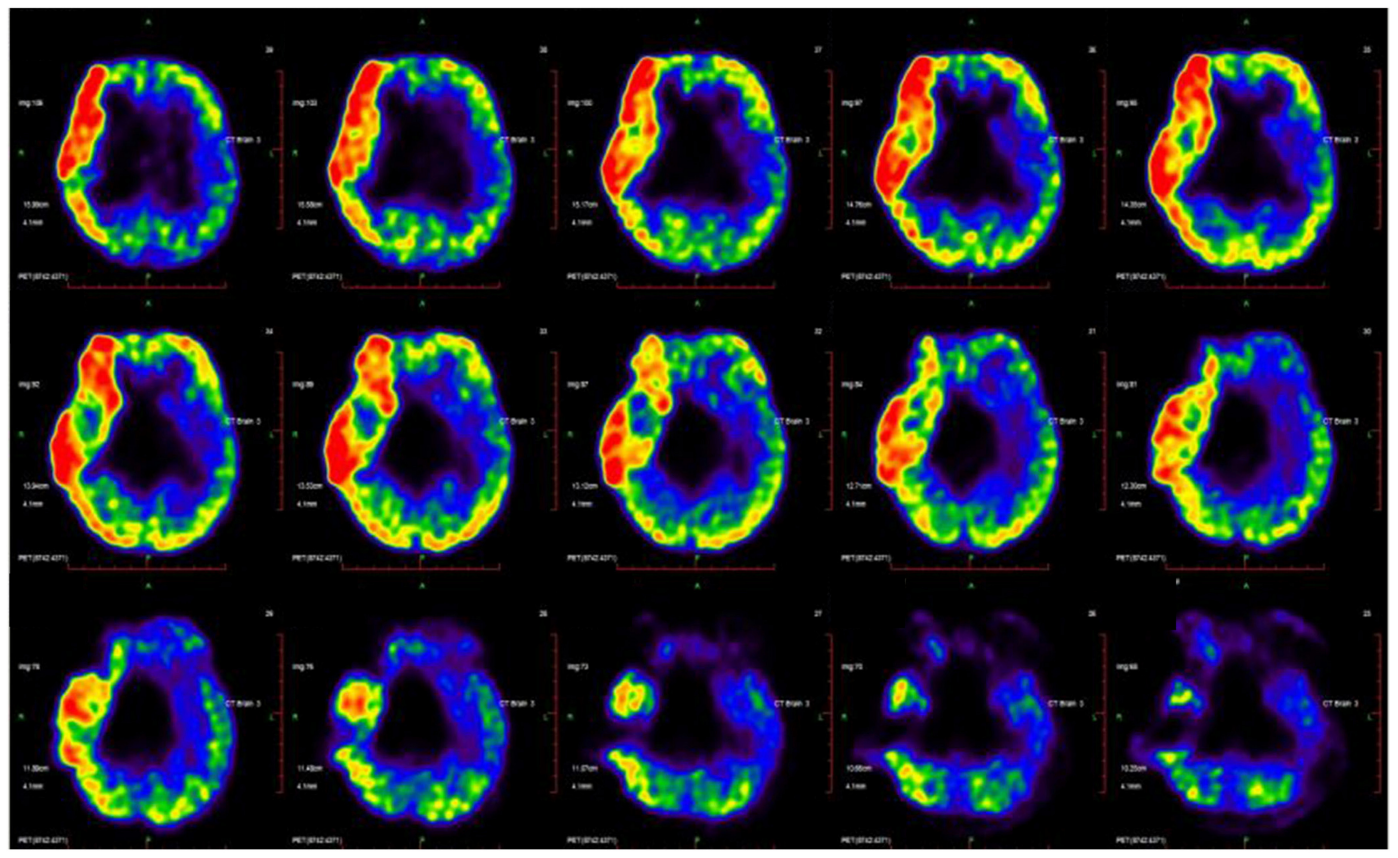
CP's disease-related pattern identified by analysis of FDG PET scans from 31 CP patients. The glucose metabolism in the left cerebellum relative higher than the right hemisphere.
